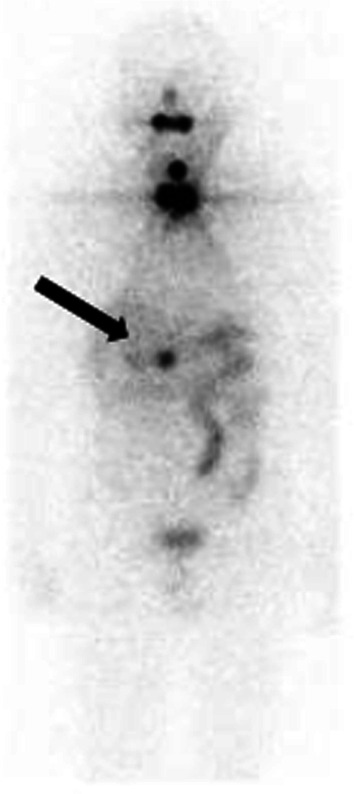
Computed tomography (CT) scan showing a small round hypodense mass (10 × 20 mm in size) adjacent to the head of the pancreas (black arrow)
